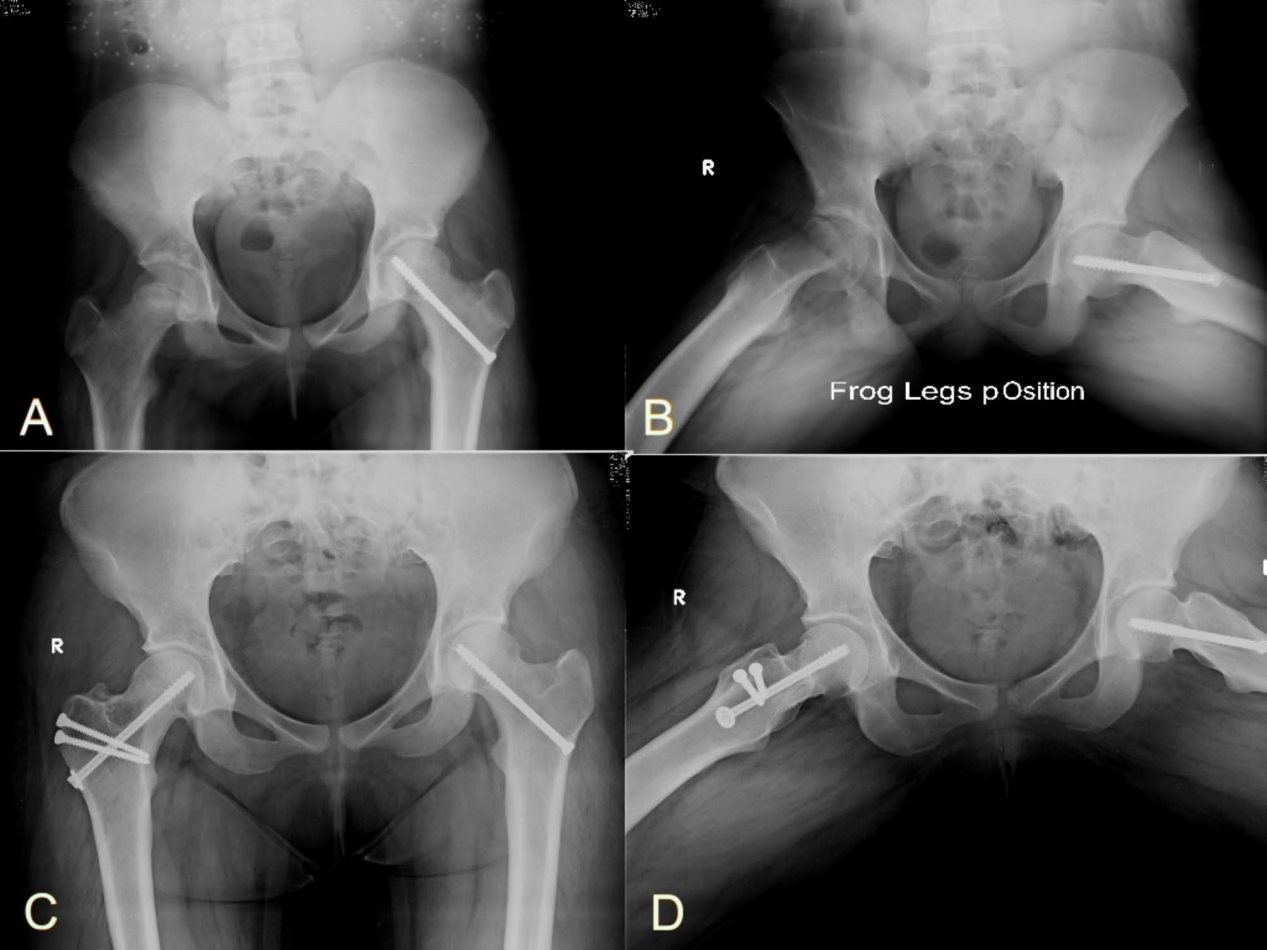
A) and B): Preoperative radiographs of a 13-year-old female patient with chronic stable SCFE. C) and D) Final follow-up x-rays done seven years after the surgery show no evidence of AVN or hip osteoarthritis with complete healing of the osteotomy site
